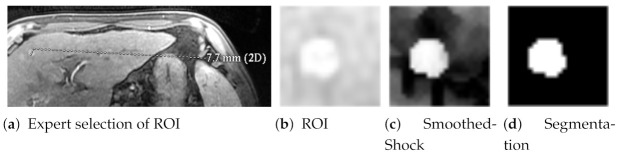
The steps of HCC segmentation from MRI slices.
